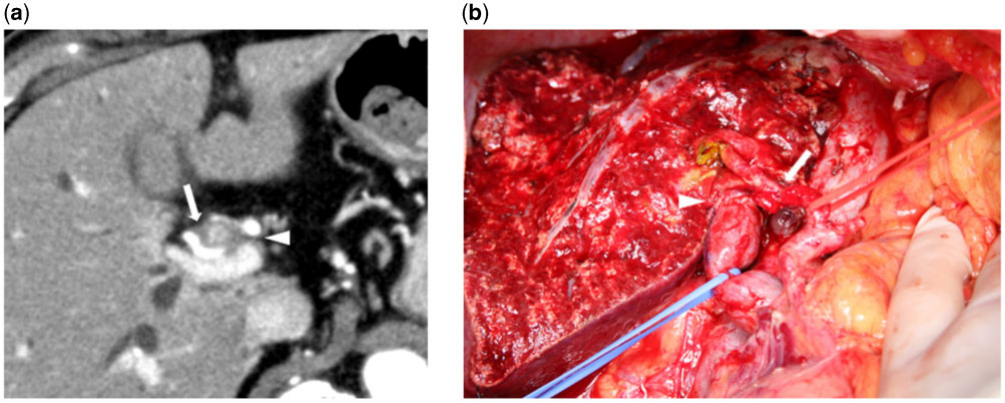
Perihilar cholangiocarcinoma a Computed tomography (axial view). The perihilar tumour is in contact with the hepatic artery (arrow) and portal vein (arrow head). b Image of left trisectionectomy, caudate lobectomy, with hepatic artery and portal vein resection and reconstruction. The right hepatic artery (arrow) and portal vein (arrow head) were reconstructed with end-to-end anastomosis
